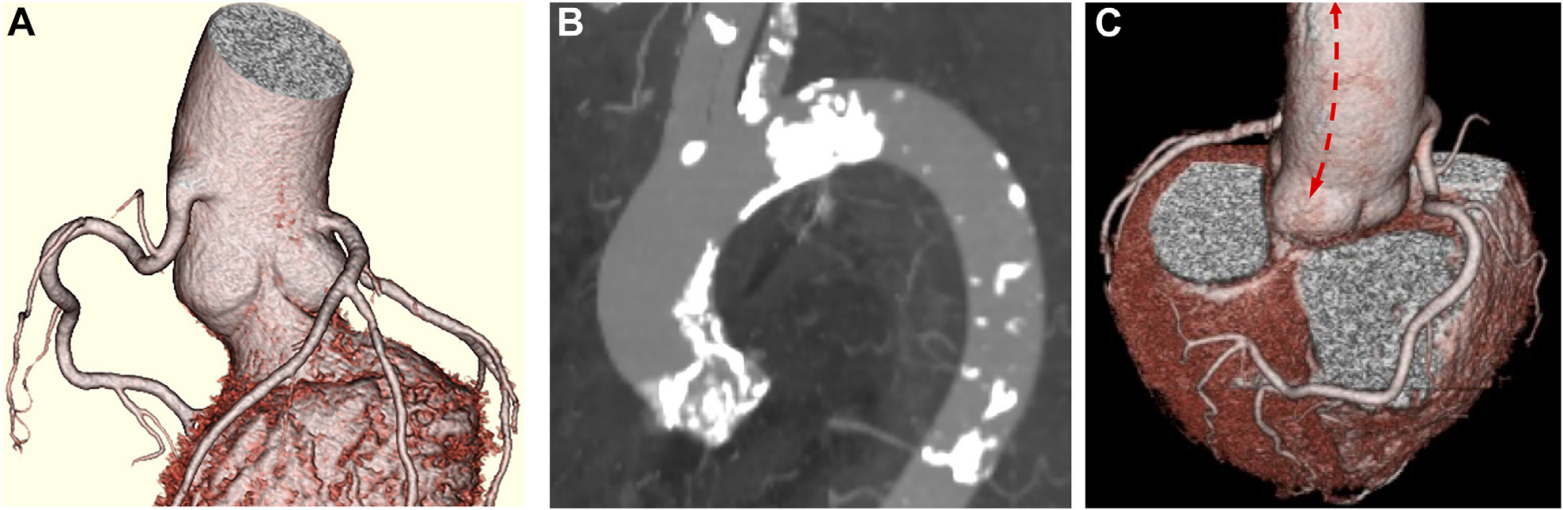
(A) Preoperative 3-dimensional reconstructed contrast-enhanced computed tomographic image showing the high takeoff of the right coronary artery in patient 1. (B) Distribution of calcification in the ascending aorta and sinutubular junction of patient 6. (C) The straight longitudinal aortotomy line (dashed red line with arrows) on a preoperative 3-dimensional reconstructed contrast-enhanced computed tomographic image of patient 1.
